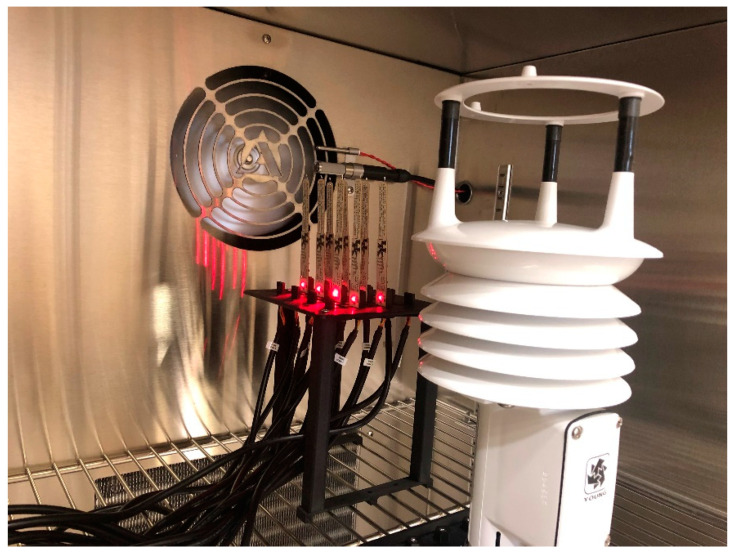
Eight PTH Probes in an environmental chamber. The environmental chamber’s temperature and relative humidity sensors and the weather station’s barometric pressure sensor were used as reference instruments for calibration and validation. The weather station’s ultrasonic anemometer also confirmed that airflow exceeded 1.0 m/s to achieve manufacturer-specified sensor response times.
